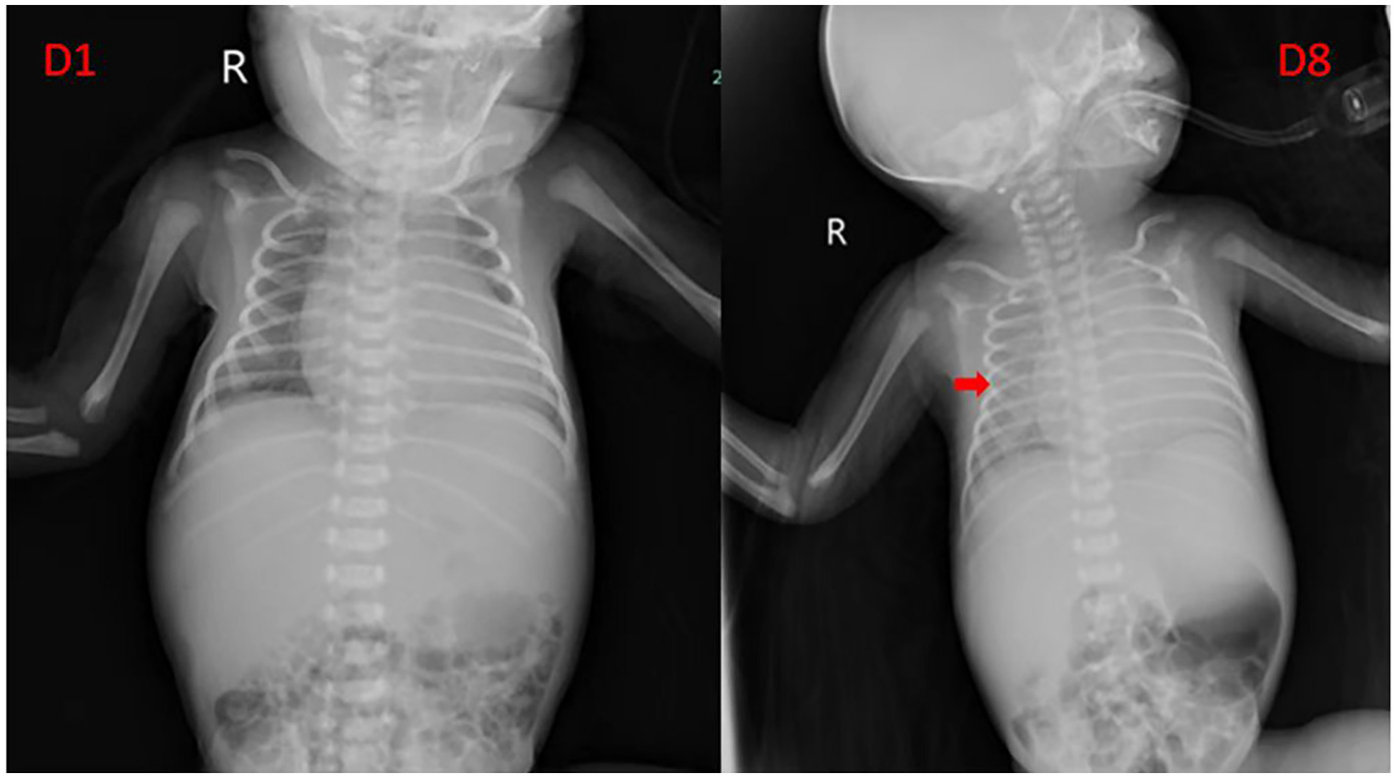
Comparison of chest radiograph before and after pharmacological treatment in case 12. The arrow indicates that the transparency of both lungs is uniformly decreased, suggesting pulmonary edema.
